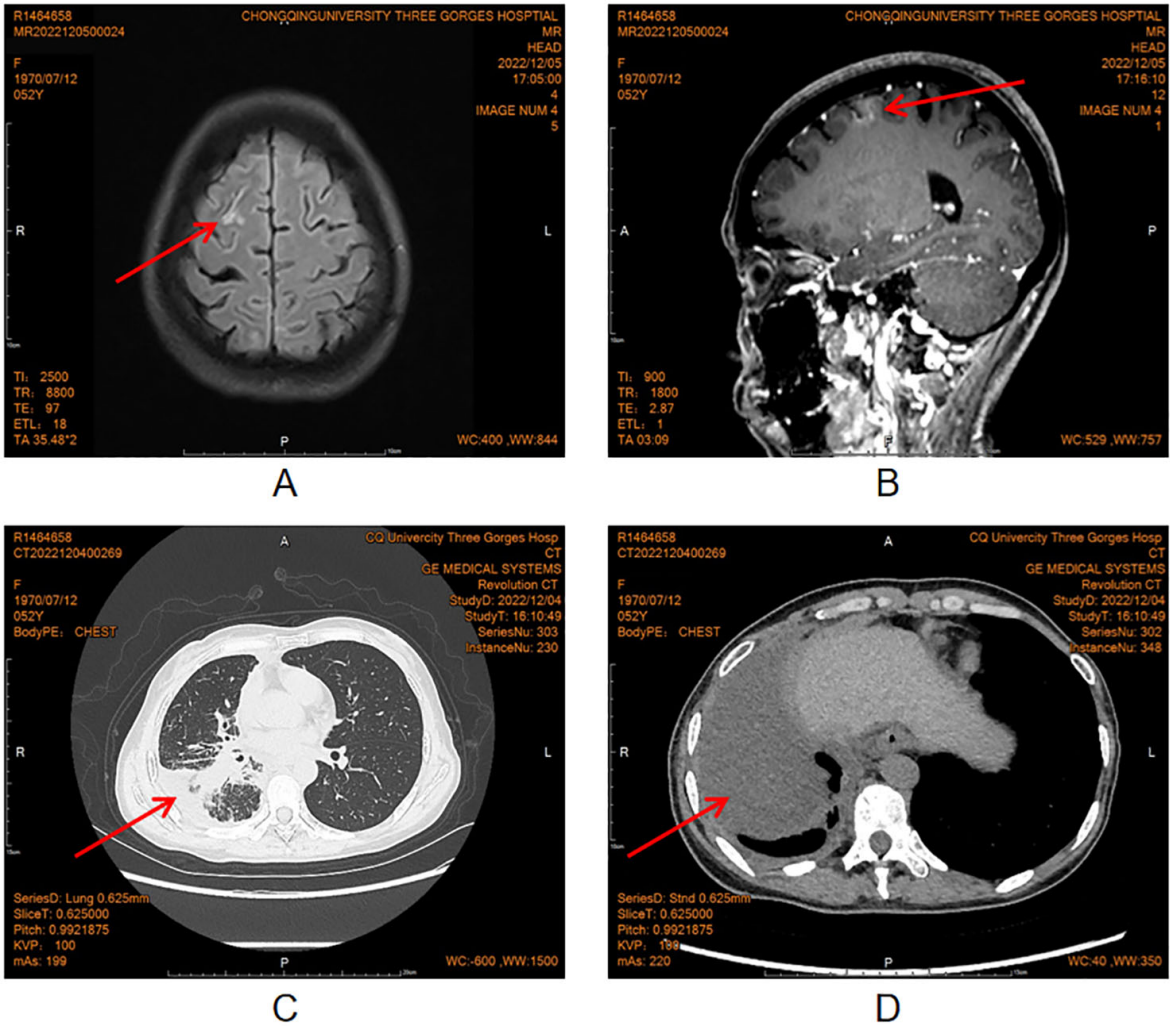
Head MRI and chest CT in December, 2022.
